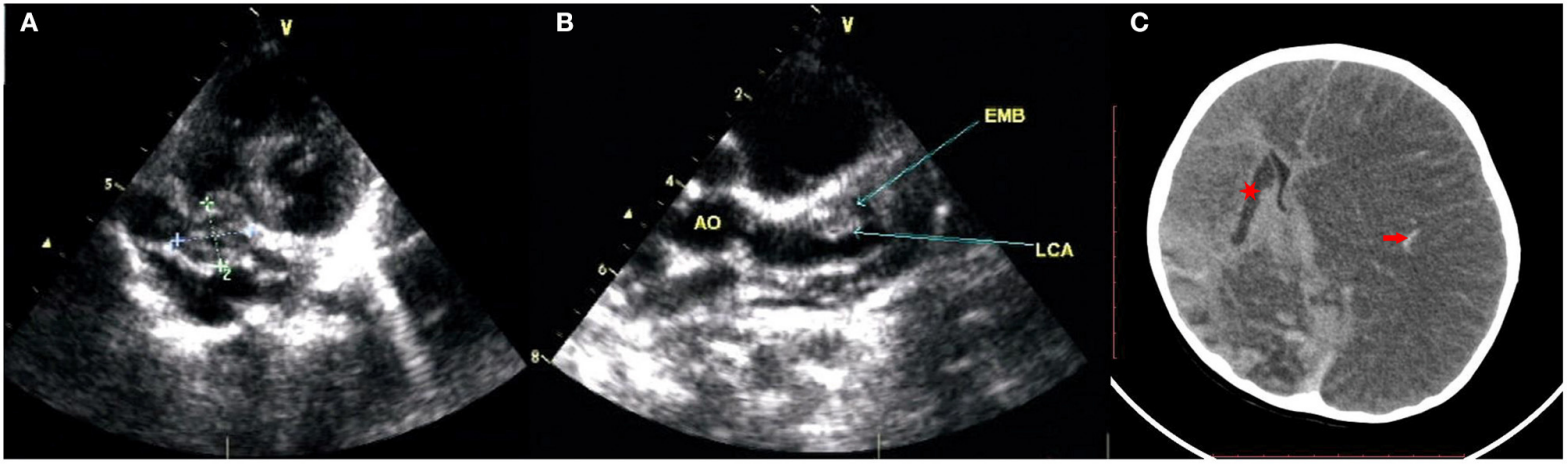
The echocardiography revealed extensive CAA and CAT. (A) Right coronary artery aneurysms sized 9 mm and thrombosis sized 19 mm × 14 mm; (B) left anterior descending artery aneurysms sized 11 mm and thrombosis sized 11 × 9 mm, left circumflex coronary artery aneurysms sized 14 mm. (C) The brain CT showed cerebral infarction of right basal ganglion (red star), hemorrhagic stroke involved in an entire left cerebral area with obviously secondary cerebral swelling, edema (red arrow).
